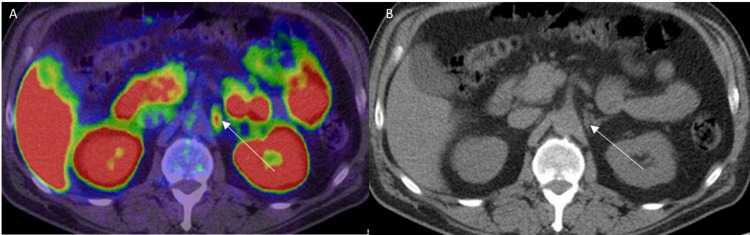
Normal PSMA uptake in the celiac ganglia A: 68Ga PSMA PET/CT axial image of the abdomen shows physiological uptake in the left celiac ganglia (white arrow). B: Correlate CT shows ovoid-shaped left celiac ganglia (white arrow).
